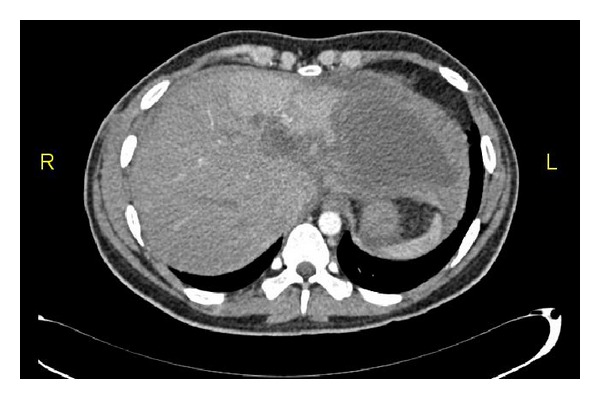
Follow-up abdomen CT (after 4 weeks of the TAE) showed start of liver regeneration and resolution of left lobe cystic changes.
